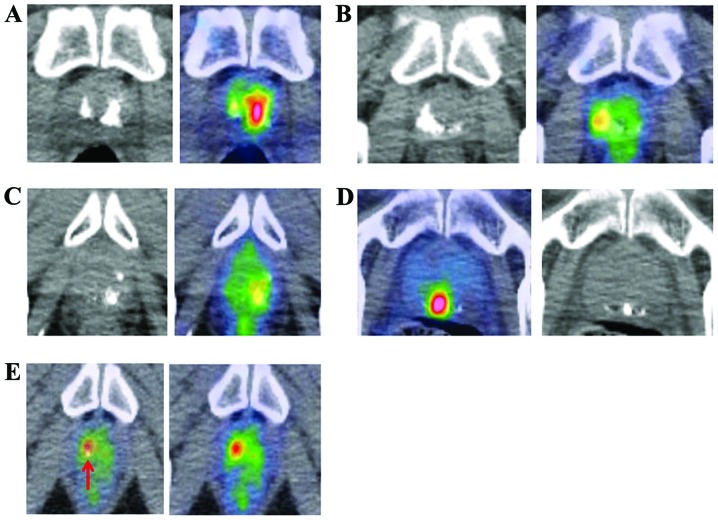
Benign uptake. All cases of FDG uptake coexisting with calcification were benign lesions irrespective of the site. (A–D) Calcification may be influenced by attenuation correction; therefore, changing window levels is needed for interpretation. Arrow shows calcification (E).
